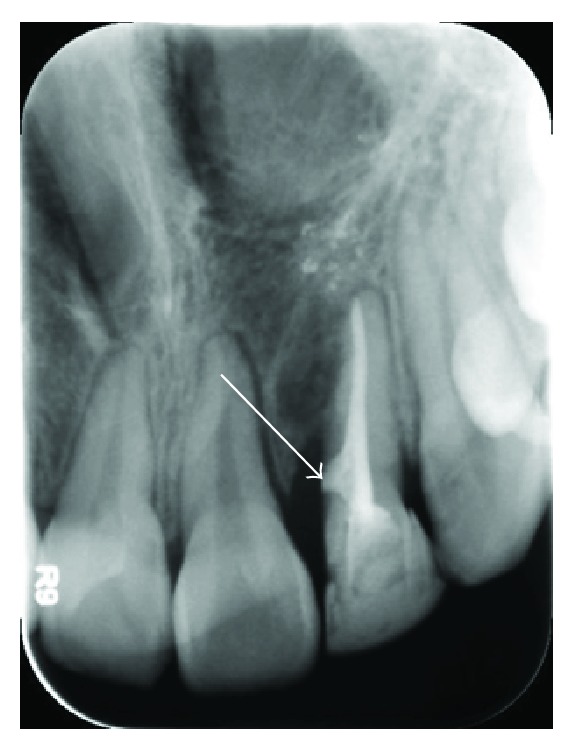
Postoperative radiograph: the defect left by the extraction of the mesial tooth is sealed with MTA.
